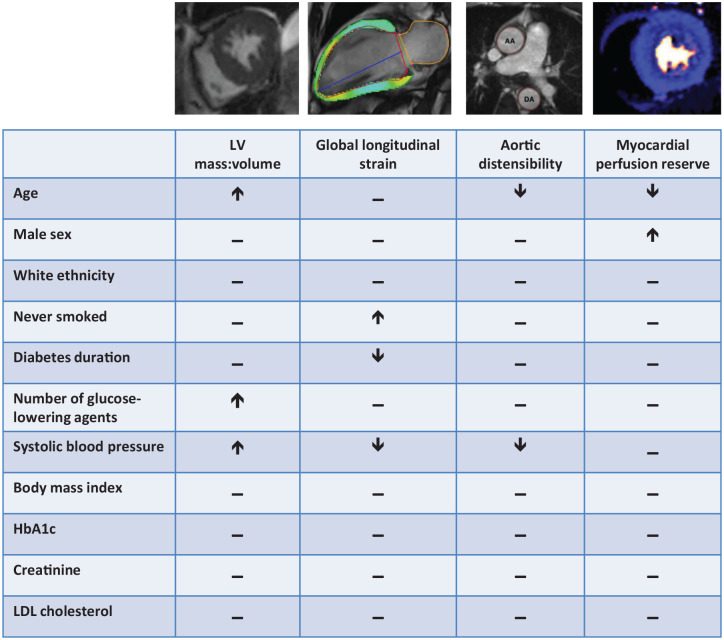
There is a high prevalence of asymptomatic SBHF in people with T2D, but the clinical contributors to early heart failure in these individuals are not clearly understood. We undertook a series of multivariable linear regression analyses in 247 adults with T2D from a multi-ethnic population with no prevalent cardiovascular disease, who underwent echocardiography and adenosine stress perfusion CMR imaging. This was to identify independent associations between clinical characteristics and markers of SBHF (concentric left ventricular remodelling, reduced global longitudinal strain, increased aortic stiffness, and impaired myocardial perfusion reserve). Age, systolic blood pressure, BMI and smoking history, but not glycaemic control, were the major determinants of SBHF. BMI, body mass index; CMR, cardiovascular magnetic resonance; SBHF, stage B heart failure; T2D, type 2 diabetes.
